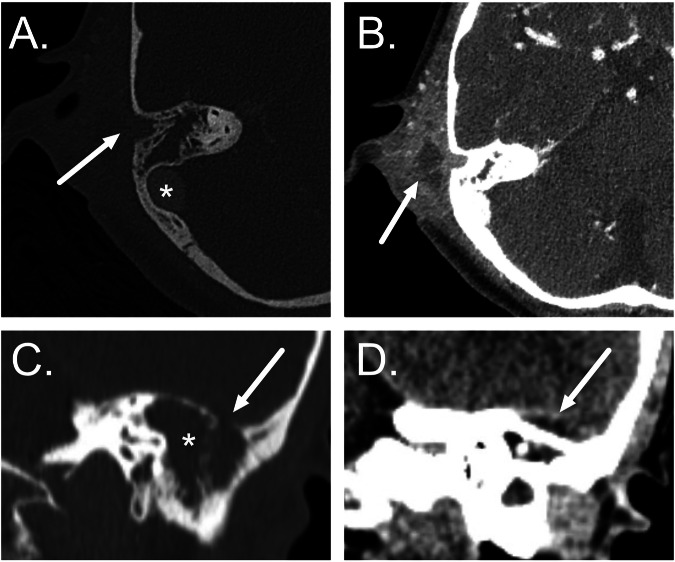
Acute coalescent mastoiditis. A, B Axial post-contrast CT images show localized erosion of the mastoid septae and lateral bony cortex (arrow in A) by acute coalescent mastoiditis associated with an adjacent post-auricular inflammatory abscess (arrow in B). Note the normally enhancing sigmoid sinus (asterisk in A) adjacent to the intact bony sigmoid plate. C, D Coronal contrast-enhanced CT images show opacified mastoid with septae destruction (asterisk in C) and a focal bony erosion at the tegmen mastoid (arrow in C) with an adjacent extradural abscess (arrow in D)
